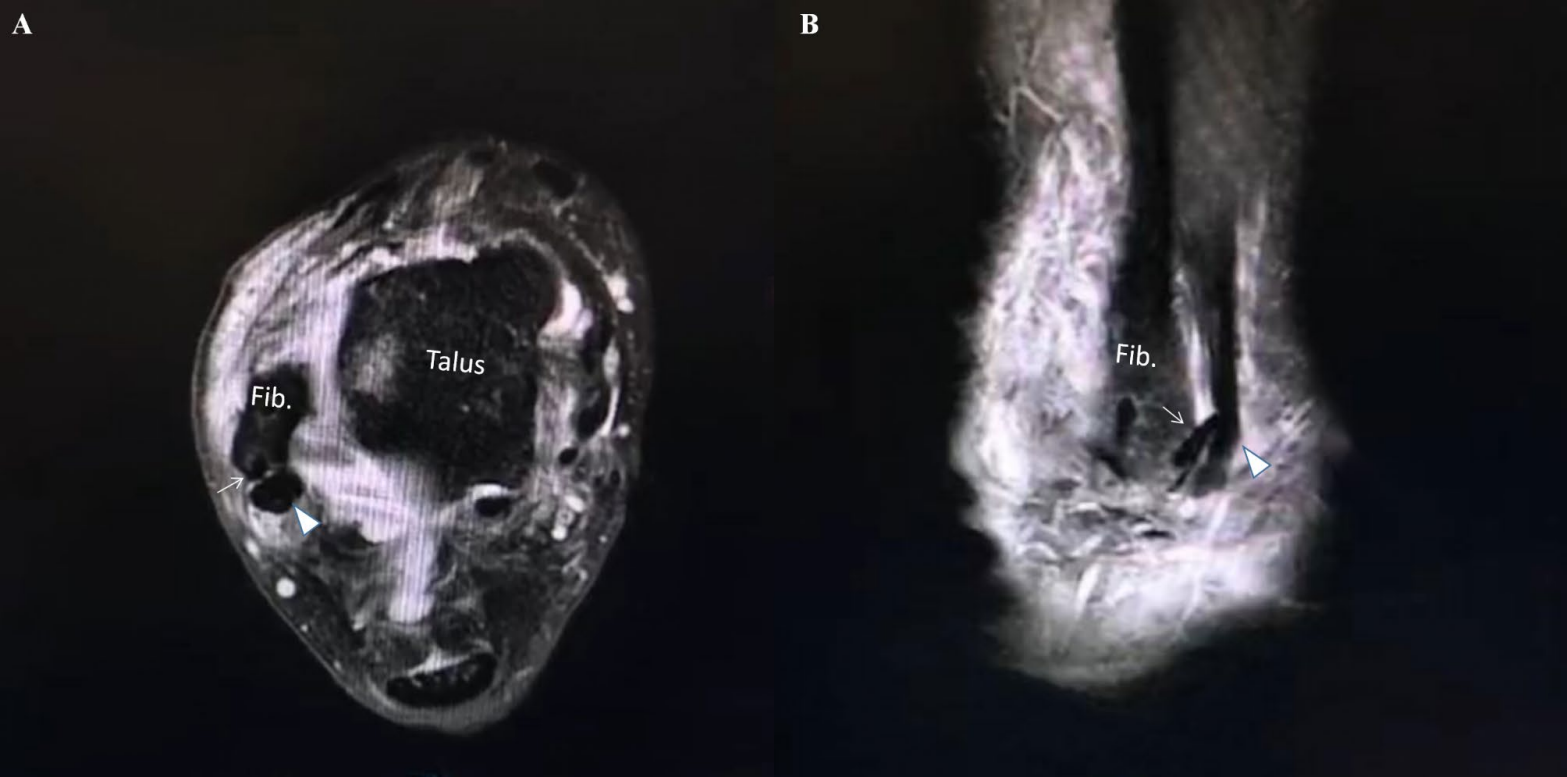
Postoperative MRI in a patient performed a arthroscopic repairing of lateral ankle ligament. A: axial view, B: sagital view. Fib.: fibule, the suture anchor (white solid arrow) penetrated through the posterior wall of the distal fibule, and disturbed the peroneal tendons (white triangle)
